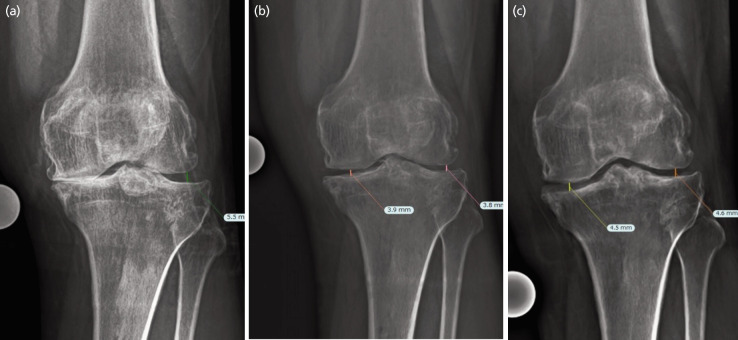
(a) pre-operative, (b) post-operative in six months, and (c) post-operative in two years of weightbearing knee radiograph series showing improvement in the joint width.
